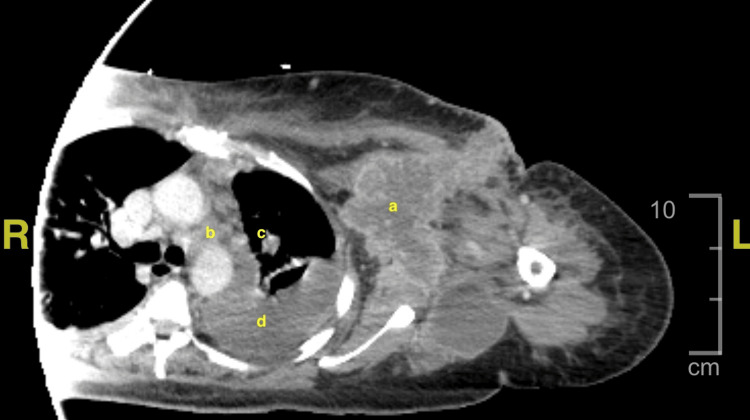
Computed tomography (CT) scan of the chest with contrast demonstrating axillary abscess, soft tissue mass, pulmonary nodule, and pleural effusion (a) left axillary abscess measuring 7.9 x 9.0 x 9.5 cm, (b) left hilar soft tissue mass measuring 3.6 cm, (c) left upper lobe pulmonary nodule measuring 1.6 cm, and (d) left pleural effusion
